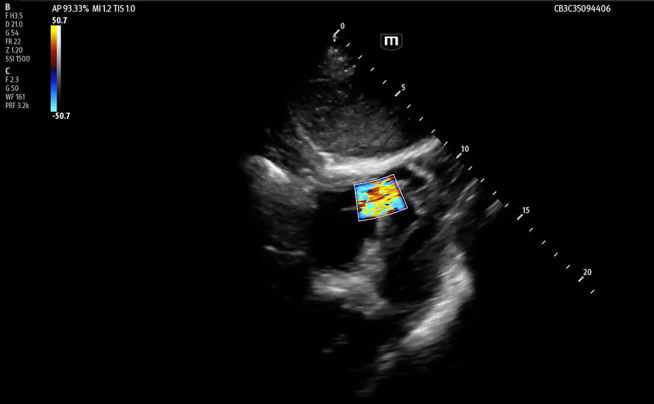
Point-of-care ultrasound demonstrating ventricular septal defect symptoms.
